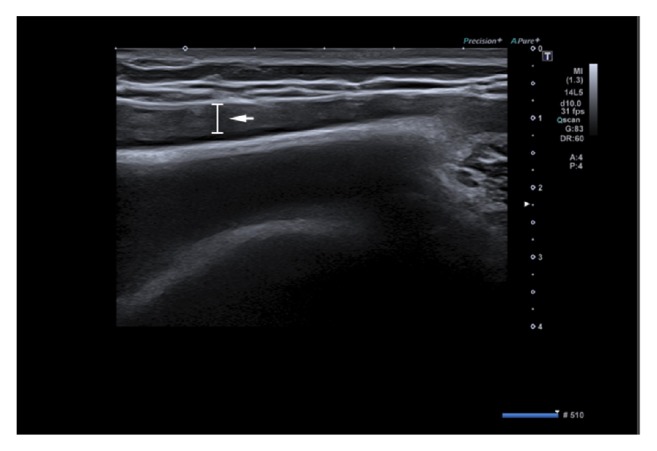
Ultrasound of the left breast demonstrates periprosthetic fluid reaction in the inner region (white arrow).
